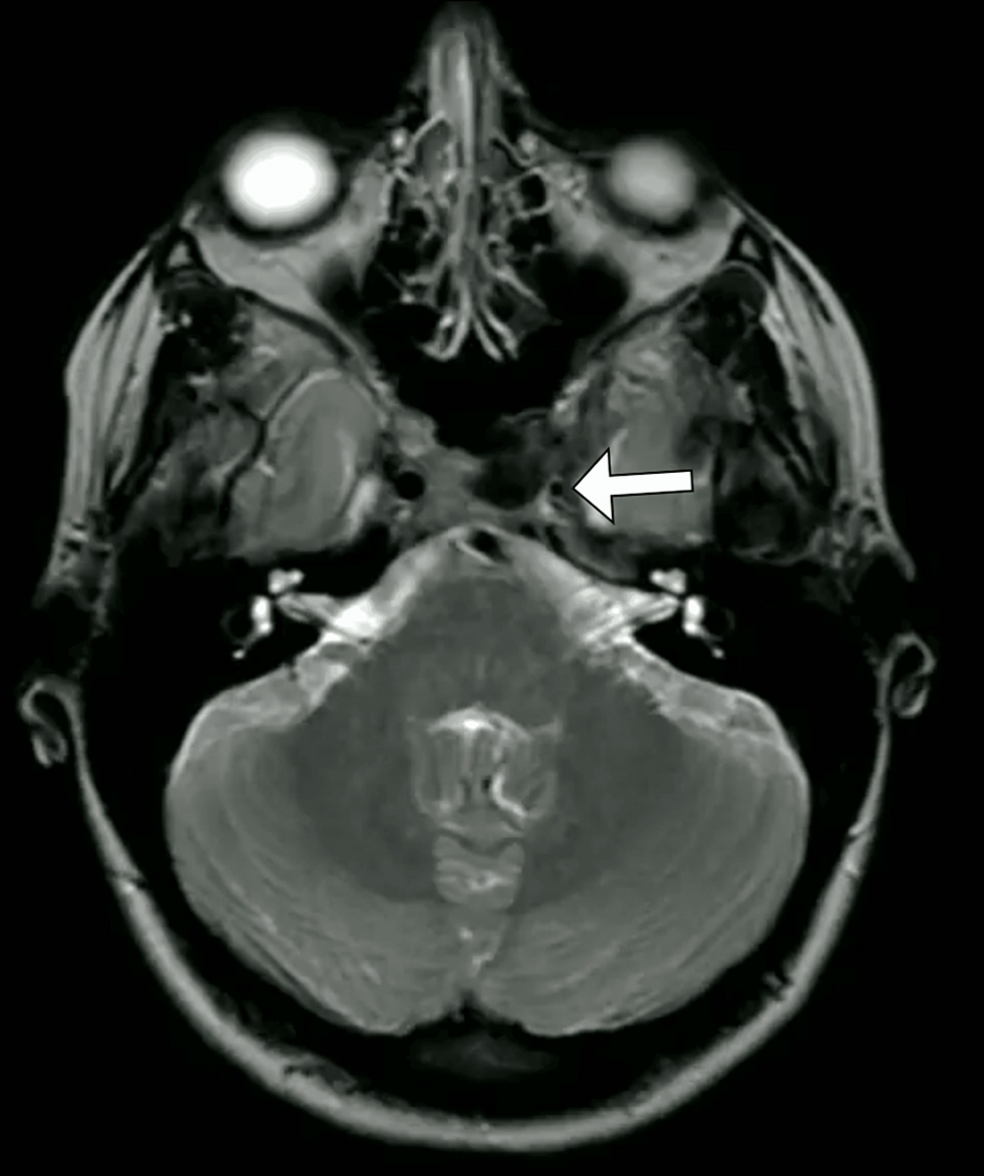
T2-weighted brain magnetic resonance image highlighting the constriction of the left internal carotid artery (arrow).
